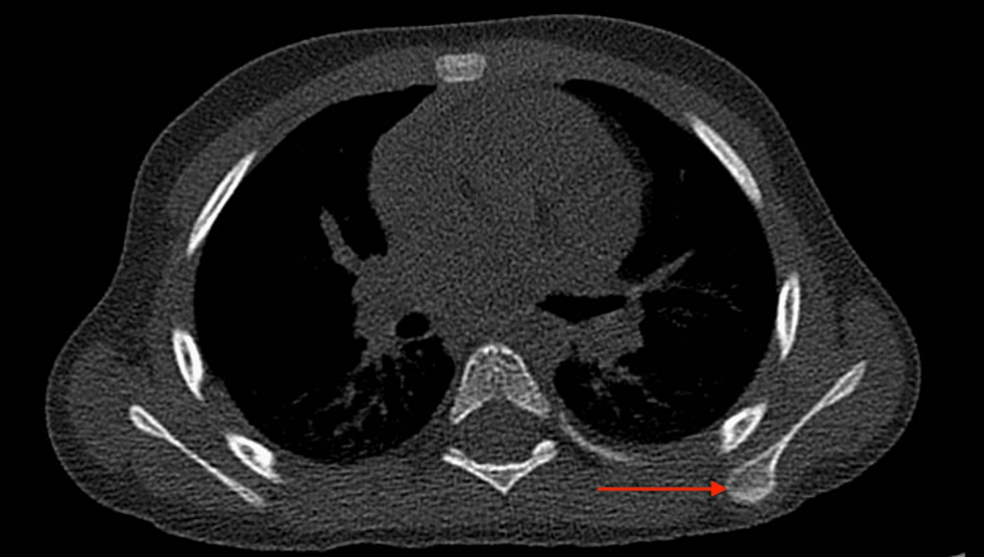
CT scan showing exostosis of the left scapula
